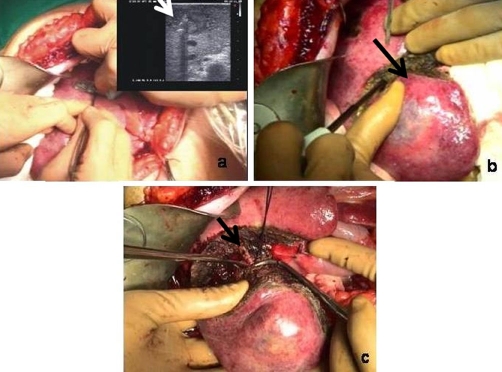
(A) Intraoperative ultrasound and RFA of the S4 lesion. (B) Segmentectomy of the S6 lesion. (C) selective
